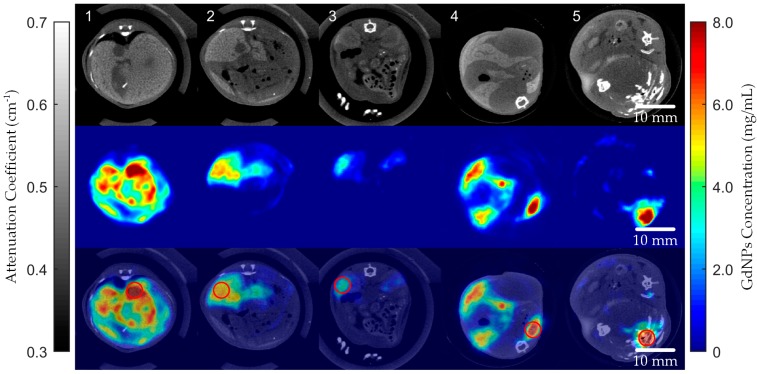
Transmission CT images, XFCT images and CT and XFCT fused images of the 5 target slices. The red circles denote the sampling points of the target regions.
